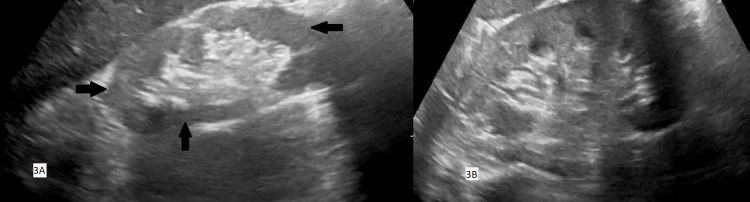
Renal ultrasound demonstrating asymmetry between kidneys (A) Right kidney ultrasound demonstrating cortical thinning, decreased corticomedullary differentiation, and increased echogenicity consistent with chronic scarring as shown by the arrows. (B) Left kidney ultrasound showing normal cortical thickness and echotexture.
